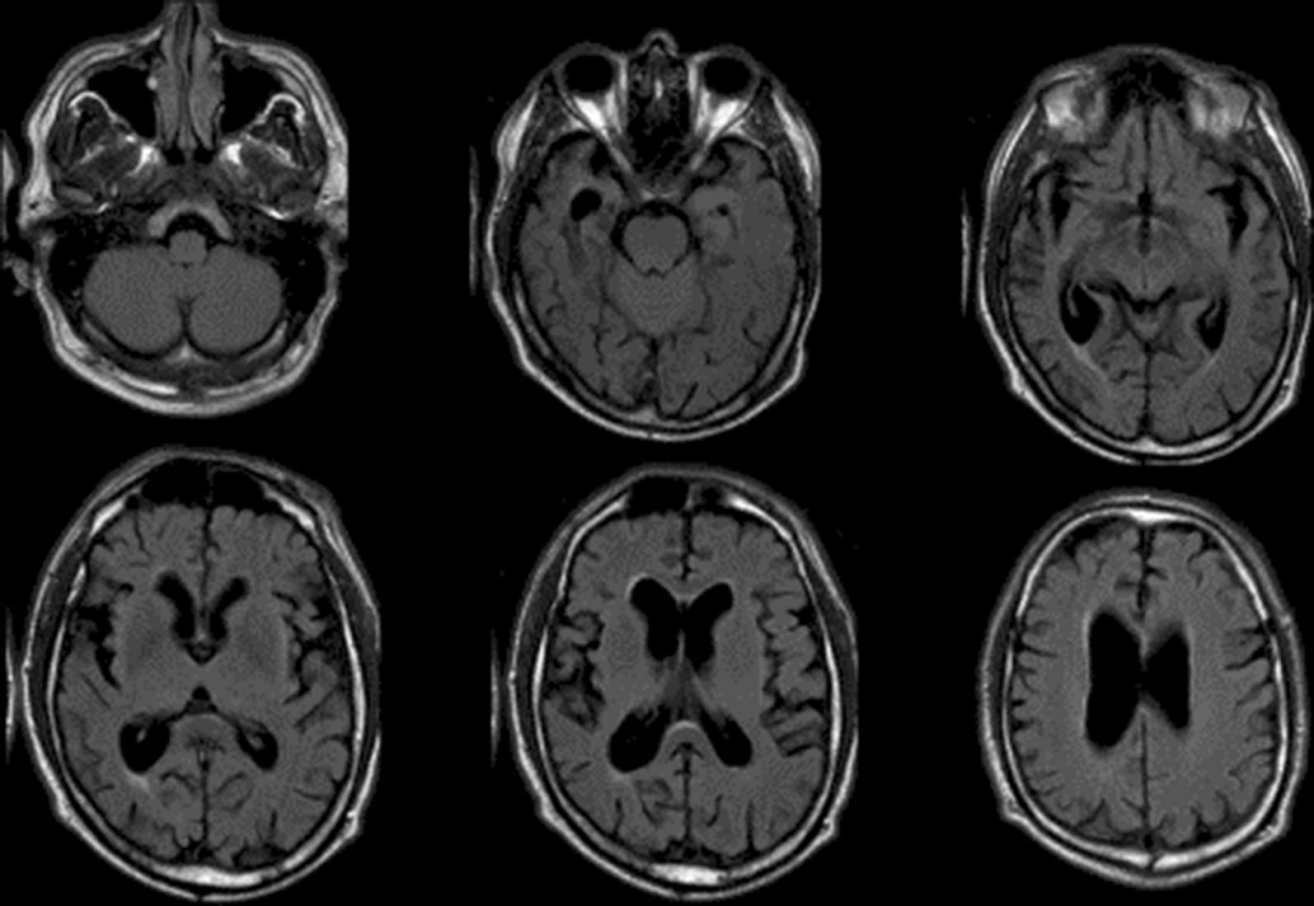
Brain axial fluid-attenuated inversion recovery (FLAIR) magnetic resonance imaging of the patient (42-year-old male) showing asymmetrical brain atrophy, more pronounced in the right hemisphere
